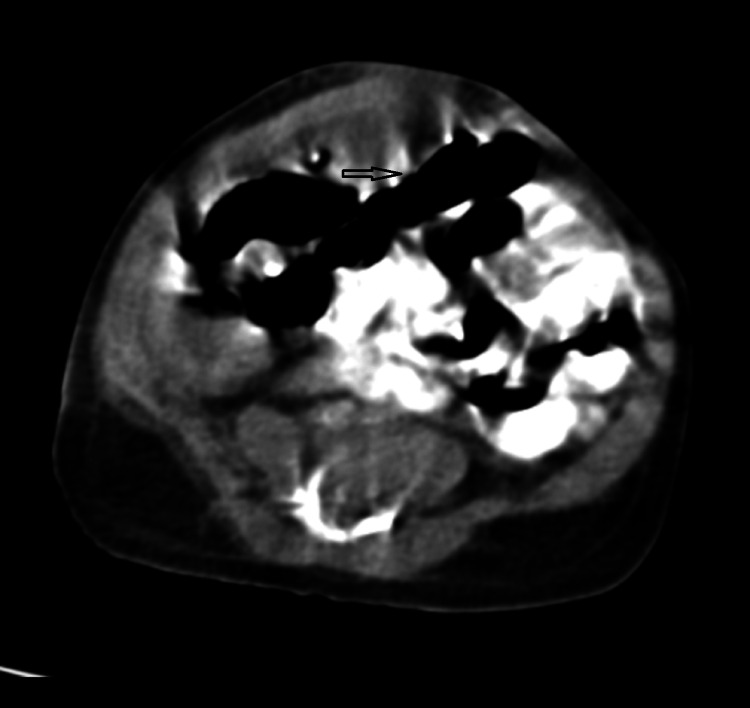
Contrast CT of the abdomen showing subtle peritoneal enhancement (arrow). CT, computed tomography
